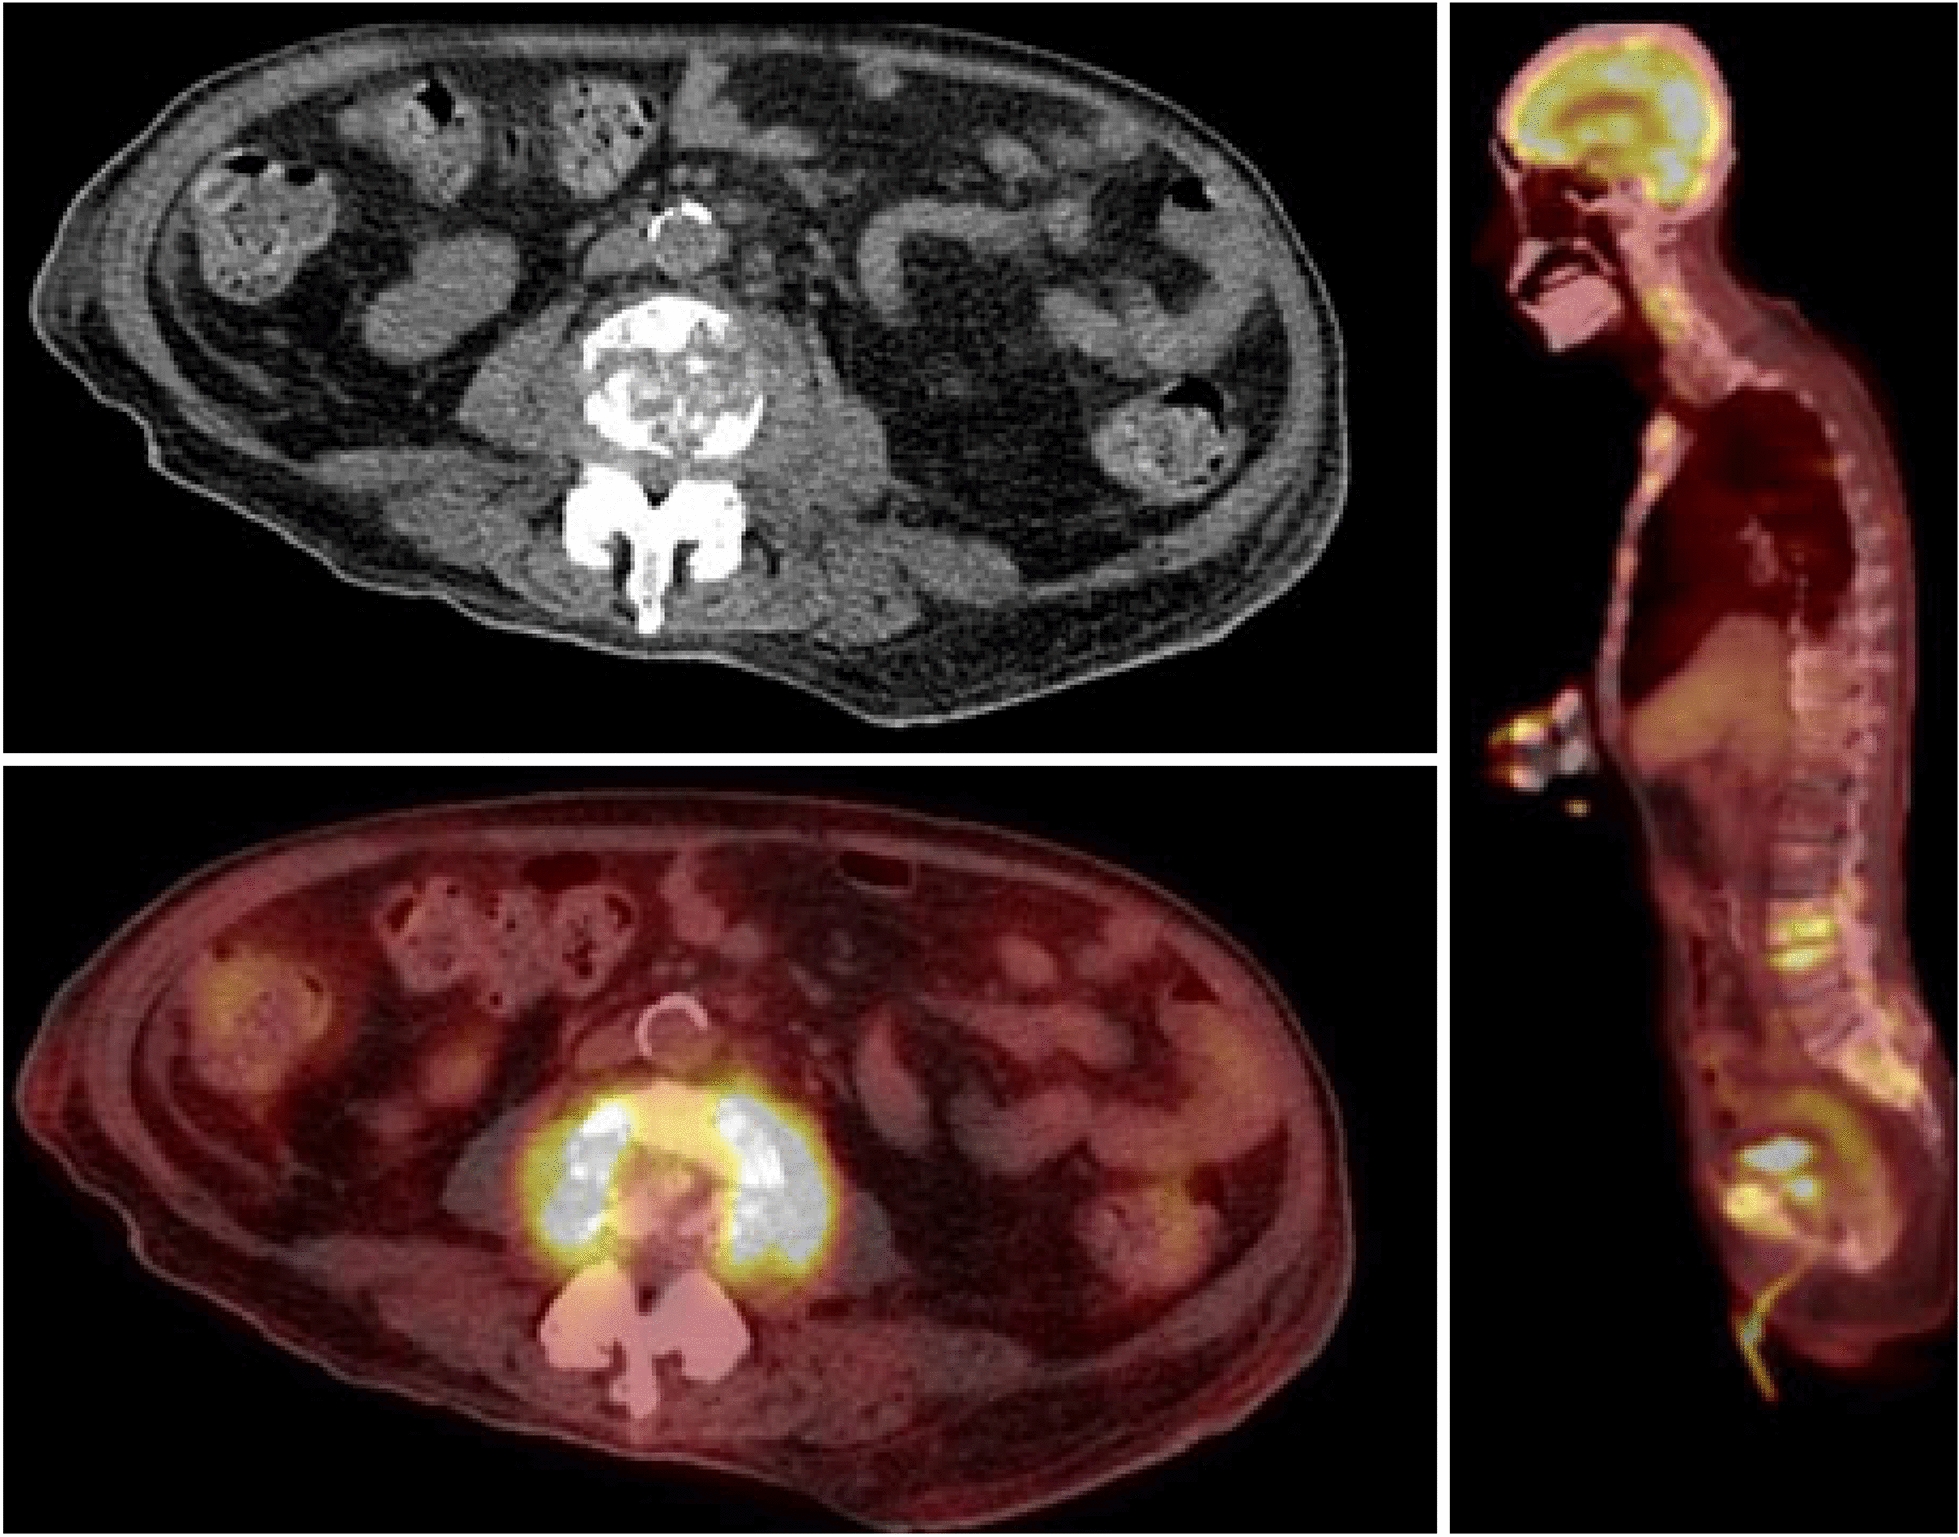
PET/CT scan 10/28/21 Multifocal hypermetabolic mixed lytic and sclerotic lesions were seen throughout the axial and appendicular skeleton. Most significantly, there were pathologic compression fractures of the L3 vertebral body with an associated hypermetabolic soft tissue mass extending into and narrowing the central spinal canal
